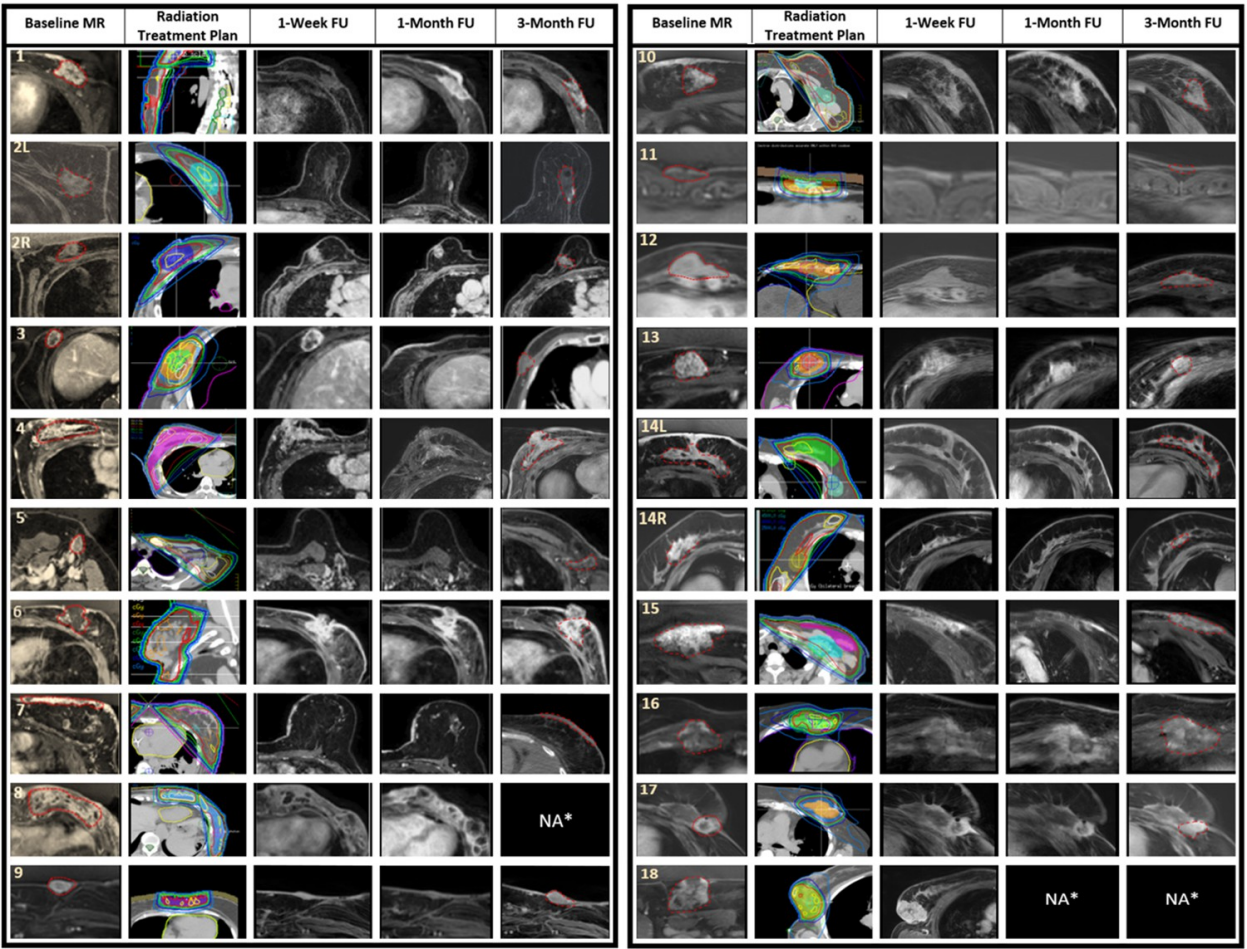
Baseline imaging and radiological follow-up at different time points. The figure details the radiation treatment plan and magnetic resonance imaging of the target tumour at baseline, 1 week, 1 month, and 3 months posttreatment. Patients were assigned numbers from 1 to 18, and for those with bilateral tumours, the left and right sides were denoted by “L” and “R,” respectively (i.e., “2L” and “2R”). The dotted red line represents the target tumour at baseline and the residual tumour or replacement fibrosis at 3 months follow-up imaging. In the radiation treatment plan, the 105% isodose line was represented in yellow, the 100% in red, the 95% in green, the 80% in dark blue, and the 50% in light blue. *Two patients died before the 3-month follow-up, represented by NA*. FU, follow-up; MR, magnetic resonance imaging; NA, not available; L, left; R, right.
